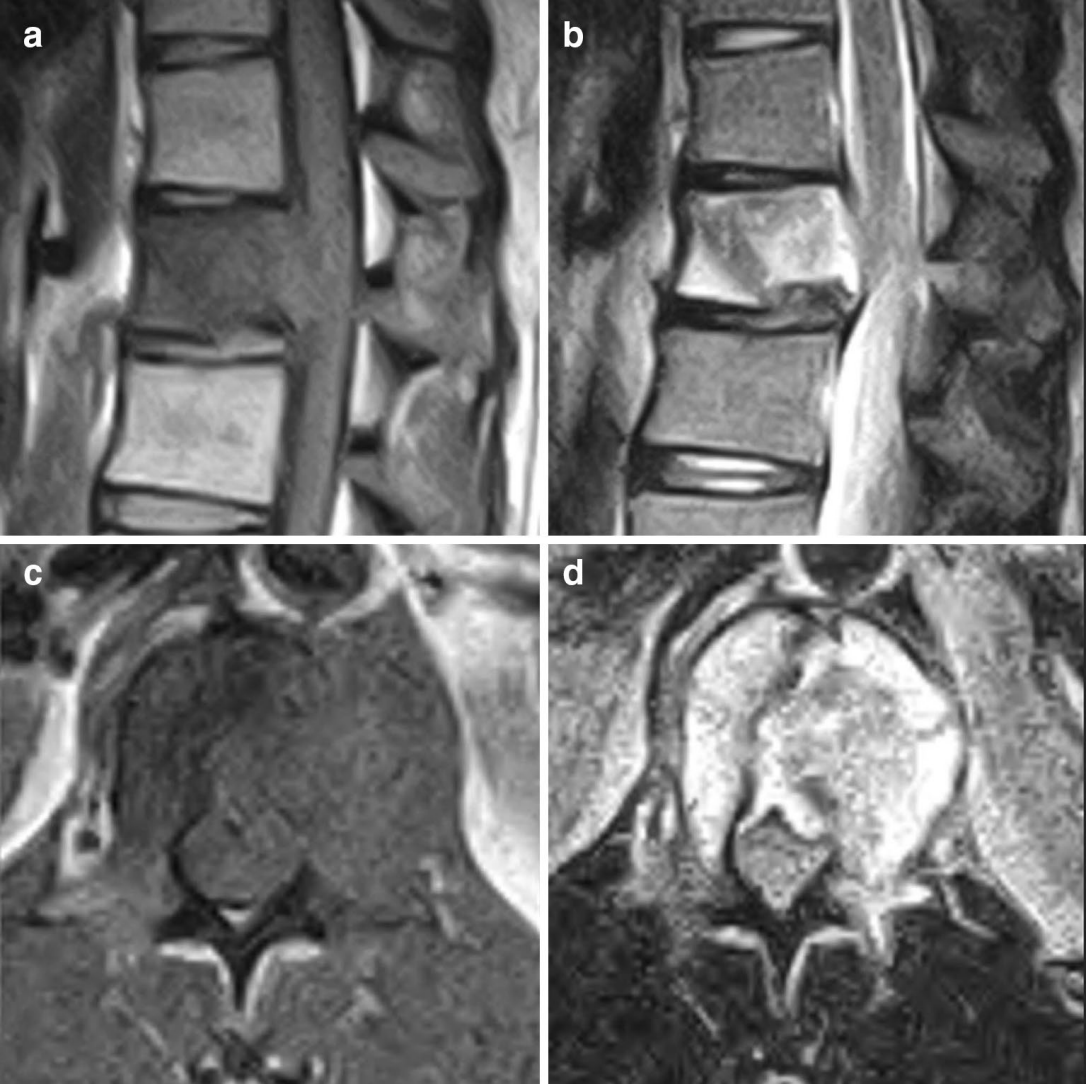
Sagittal magnetic resonance images showing low signal intensity changes of the L1 vertebral body on T1-weighted images (a) and high signal intensity changes on T2-weighted images (b). Axial magnetic resonance images showing low signal intensity changes of the L1 vertebral body and pedicle on T1-weighted images (c) and high signal intensity changes on T2-weighted images (d)
